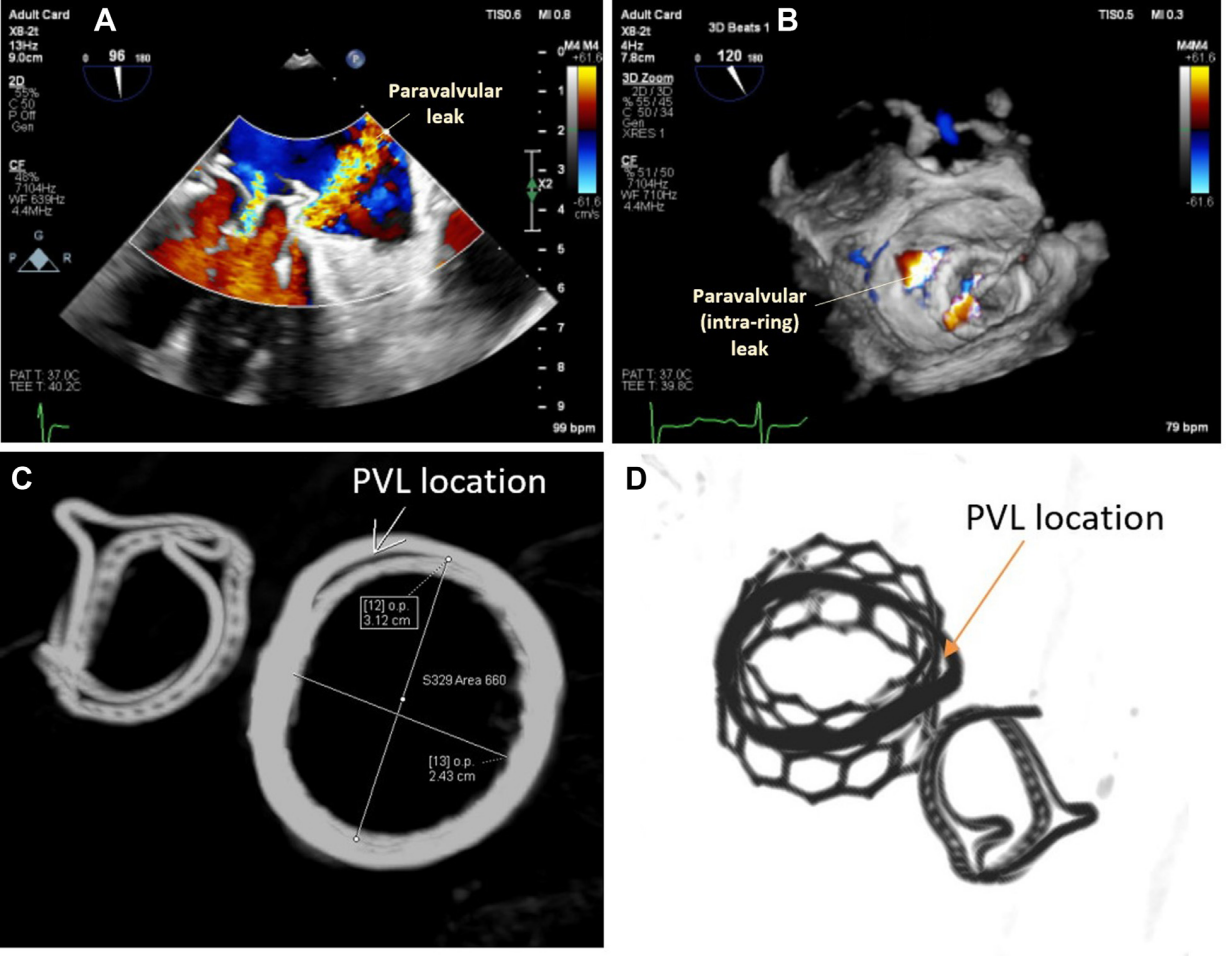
Paravalvular (Intra-Ring) Leak Between the THV and the Annuloplasty Ring (A and B) Transesophageal echocardiography and (C and D) computed tomography. PVL = paravalvular leak.
